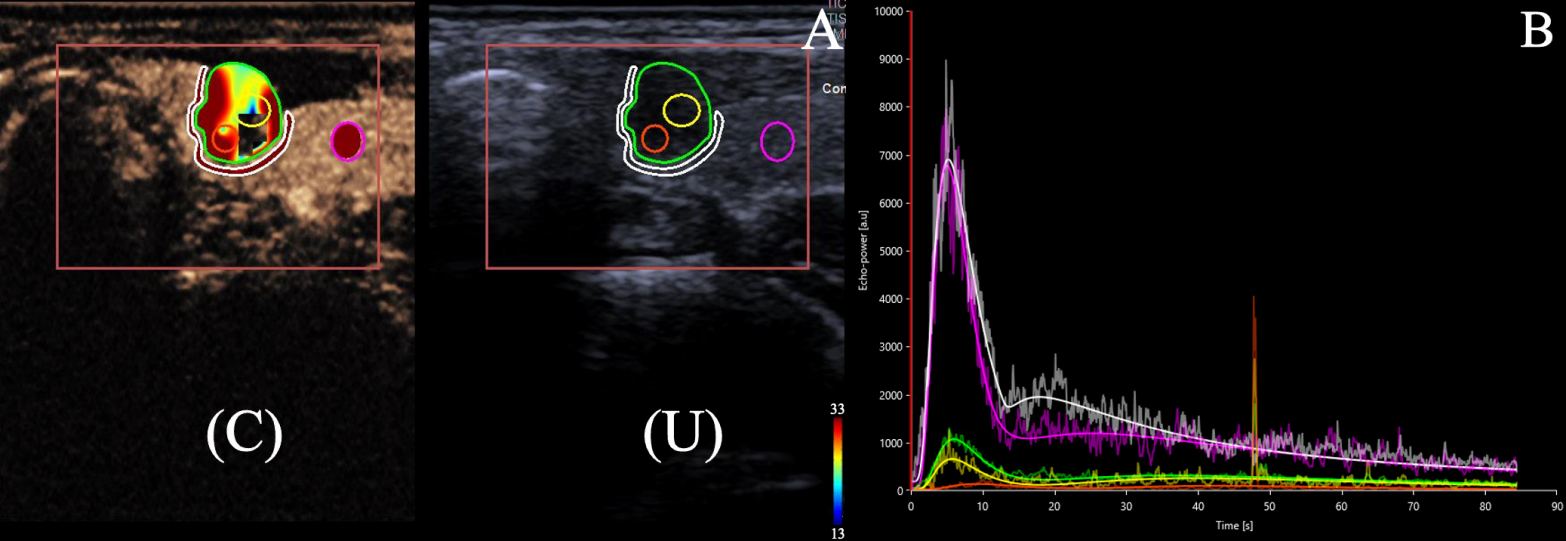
(A) 22-year-old female with a 6.7 × 5.9 × 7.0 mm nodule on the left thyroid lobe. The nodule has solid component with hypoechoic and blurred edge, which was categorized as C-TIRADS 4b. Surgical pathology showed a papillary thyroid carcinoma. (A) Dual US and CEUS image of the internal tissue of thyroid nodule (ROI 1, green circle outlining the entirety of the nodule, ROI 2 and 3, yellow and orange circles randomly selected to represent the internodular contrast-enhanced areas), the peripheral tissue of thyroid nodule(ROI 4, white circle surrounding the nodule),and the healthy tissue of the gland (ROI 5, pink circle set in the healthy thyroid tissue) under CEUS (C) and conventional ultrasound (U). (B). Time-intensity curve (TIC)s for the internal tissue (green, yellow, and orange lines), the peripheral tissue of the thyroid nodule (white line) and the healthy tissue of the gland (pink line) were generated. The lesion ROI curves were lower than the reference ROI curves, indicating hypoperfusion.
